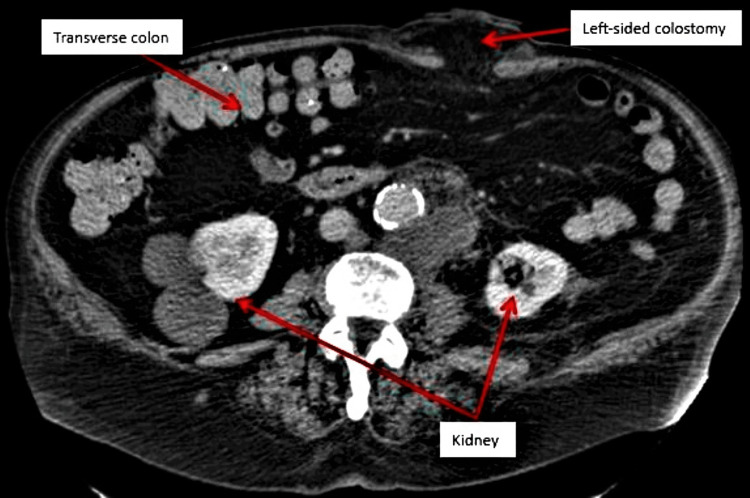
Abdominal CT after APR, colostomy creation, and chemotherapy CT, computed tomography; APR, abdominoperineal resection
